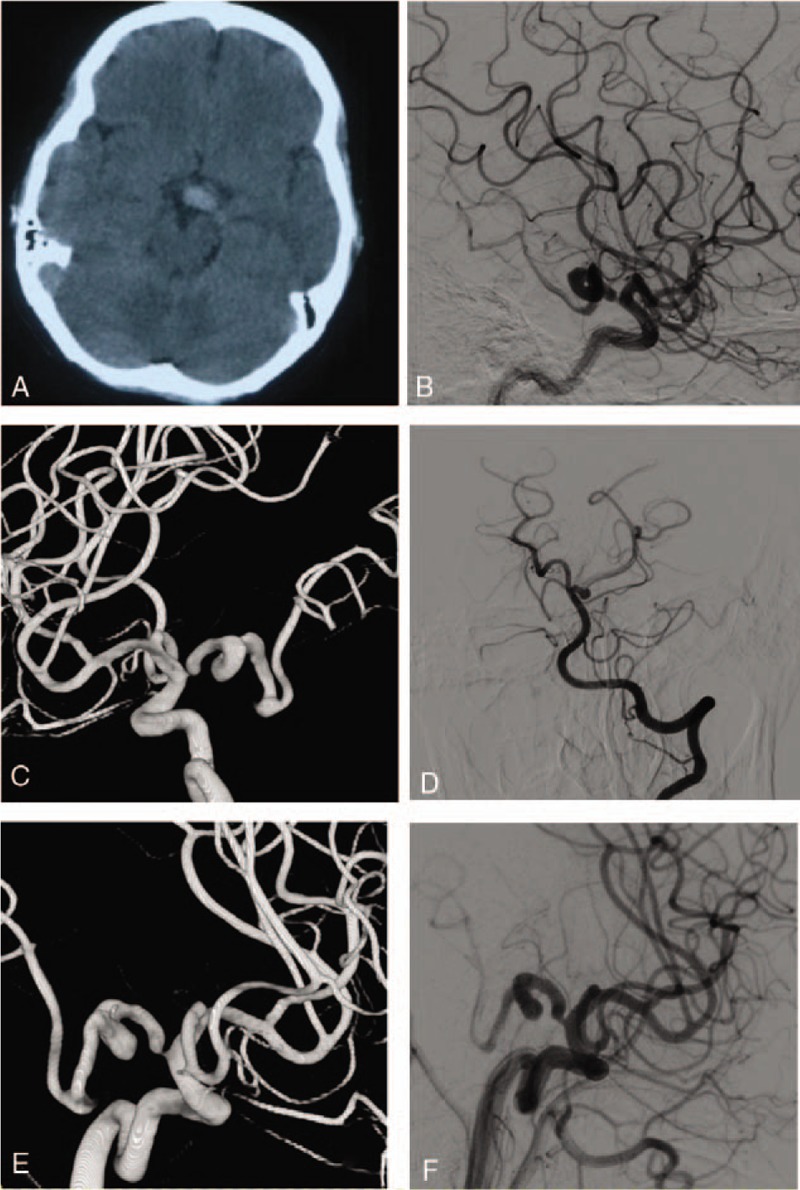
Images of the patient 1. A: Head CT showing high density shade in front of mesencephalon; B: Left ICA injection angiograms showing Pure arterial malformation of LPCOA and PCA which is moderately dilated and has a loose coil-like loop; C: Left ICA 3D injection angiograms; D: Left VA injection angiograms showing Left PCA P1 segment clear; EF: 6 mo FU.
